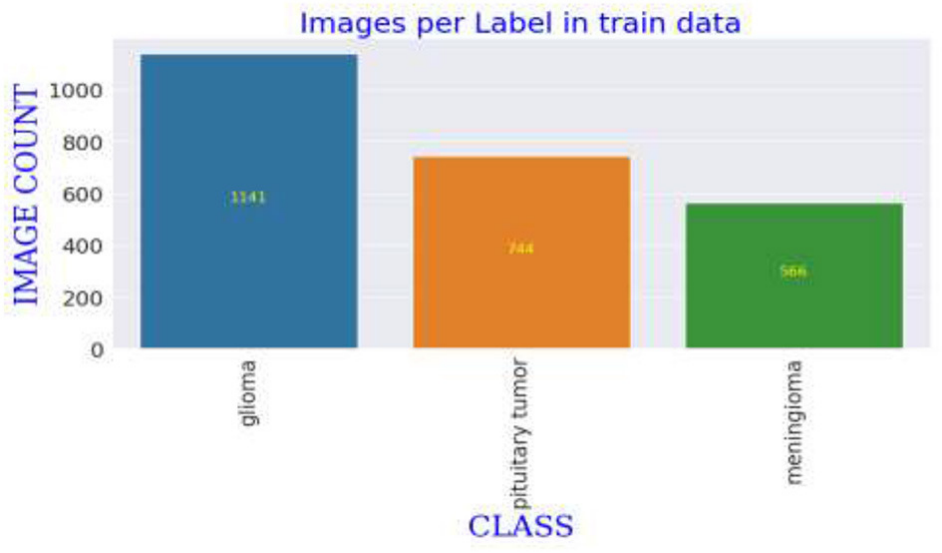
Distribution of MRI scan images across tumor types in training dataset.
